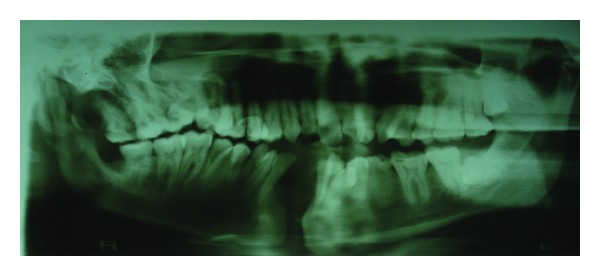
Preoperative orthopantomogram shows mandibular symphysis cleft.
